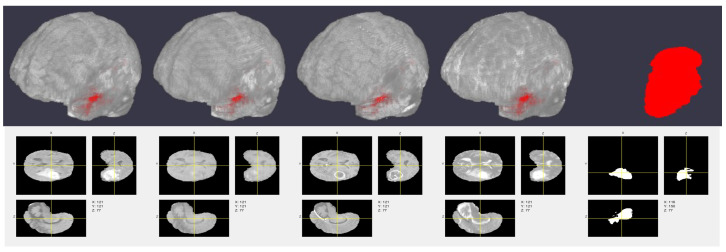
Example 3-D images and labels. From left to right: T1-weighted, T2-weighted, contrast-enhanced (CE)-T1-weighted, FLAIR, and labels. Corresponding orthogonal slices are shown in the second row.
